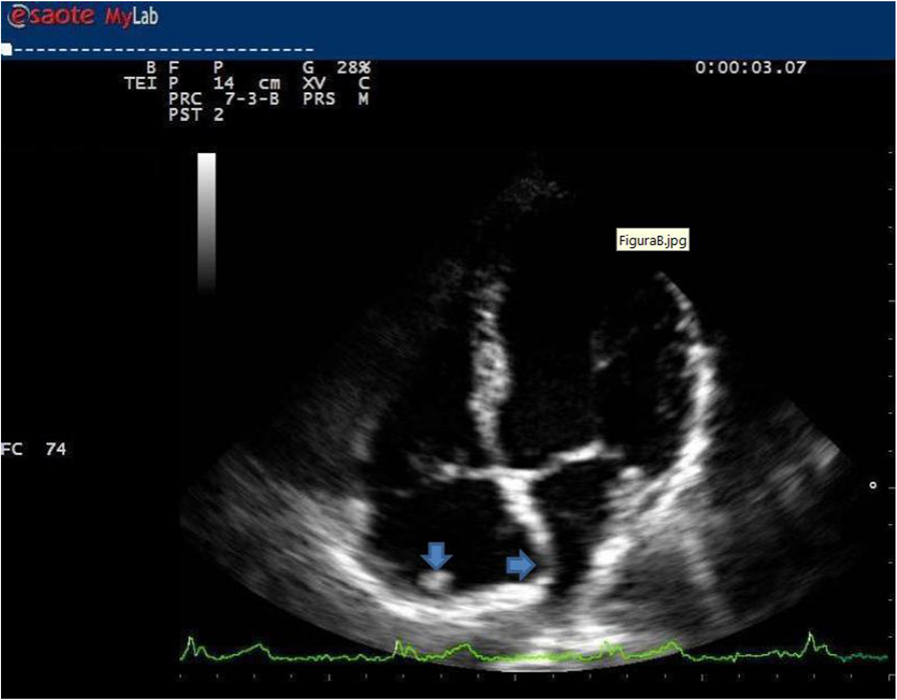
Transthoracic echocardiogram, in four-chamber apical view, shows prominent crista terminalis and atrial septal aneurysm (arrowheads) during atrial diastole.
